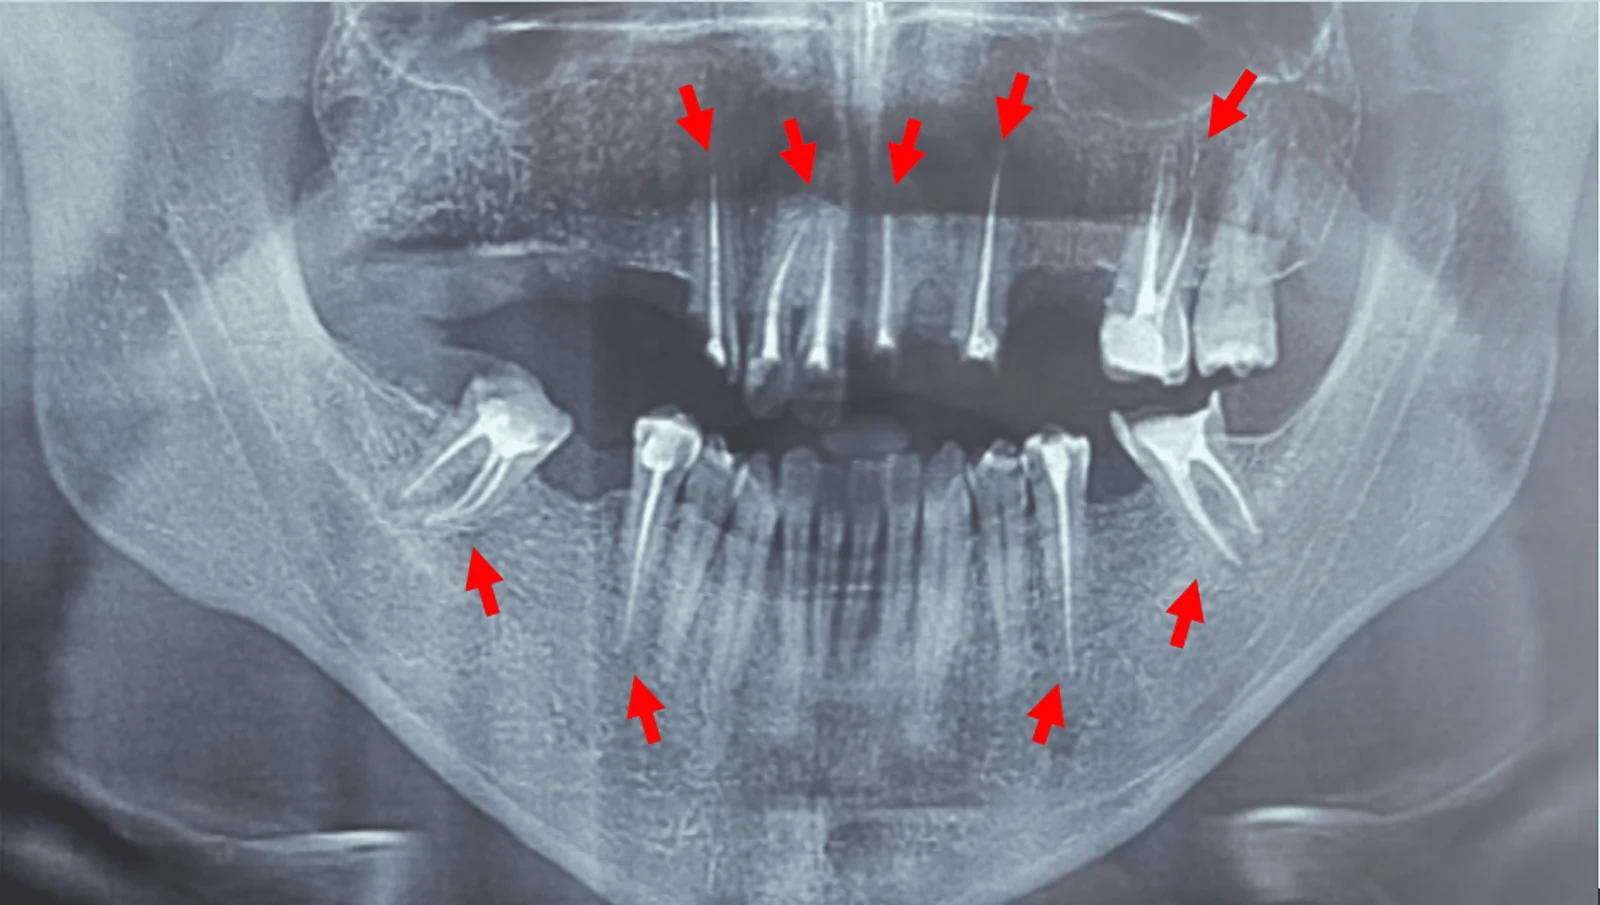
Panoramic radiograph; the red arrows show the future prosthetic abutment teeth treated endodontically
